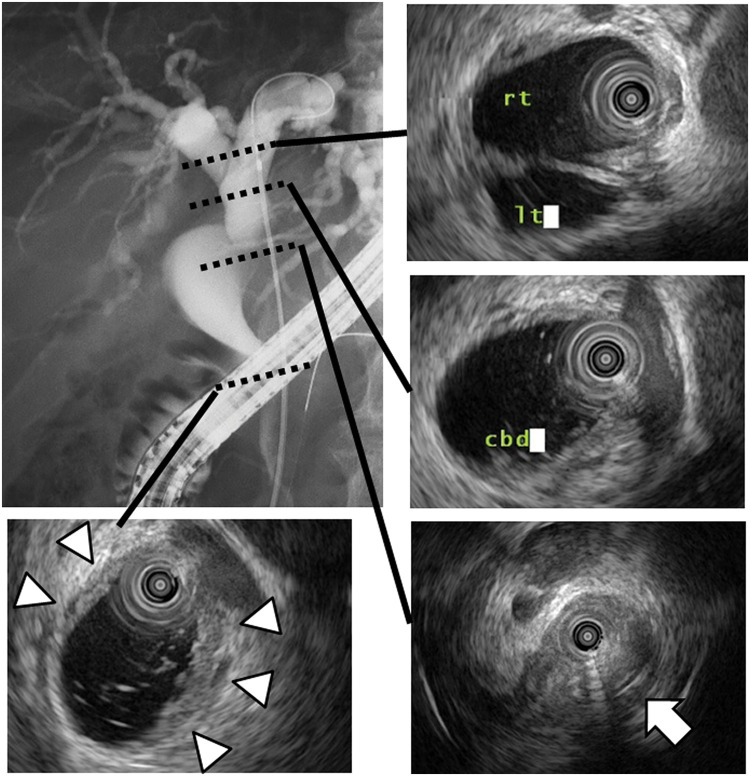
Endoscopic and ultrasonographic tumor assessments. Endoscopic retrograde cholangiopancreatography and intraductal ultrasonography displaying infiltration of the tumor from the common hepatic duct into the distal bile duct. Focal wall thickness is observed in the common hepatic duct (arrowheads), and the distal bile duct is obstructed by the tumor (arrow).
